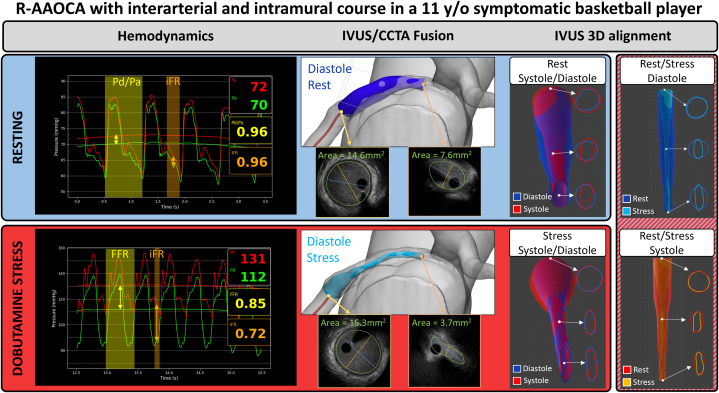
R-AAOCA With Interarterial and Intramural Course in an 11-Year-Old Symptomatic Basketball Player (Top Left, blue background) Patient-specific invasive findings at rest demonstrate a Pd/Pa ratio of 0.96 and an iFR of 0.96. Diastolic IVUS reveals a vessel with a minimal lumen area of 6.6 mm^2^ during diastole (distal reference: 14.6 mm^2^), with the 3D reconstruction coregistered to the CCTA. The vessel displays significant geometrical changes between diastole and systole. (Bottom Left, red background) Under dobutamine stress, invasive findings show a hemodynamically nonsignificant FFR_Dobutamine_ of 0.85, contrasted by a significantly lower iFR_Dobutamine_ of 0.72. IVUS shows a marked reduction in lumen area to 3.7 mm^2^ (distal reference: 15.3 mm^2^). (Right Side, red/blue striped background) Comparative analysis from rest to stress indicates that luminal deformation is significantly more pronounced during the diastolic phase than the systolic phase. This phase-specific dynamic likely explains the discrepancy between the cycle-averaged FFR_Dobutamine_ and the diastolic-specific iFR_Dobutamine_. 3D = three-dimensional; CCTA = coronary computed tomography angiography; FFR = fractional flow reserve; iFR = instantaneous wave-free ratio; IVUS = intravascular ultrasound; MLA = minimal lumen area; Pd/Pa = distal coronary artery pressure/aortic pressure; R-AAOCA = anomalous aortic origin of a right coronary artery.
